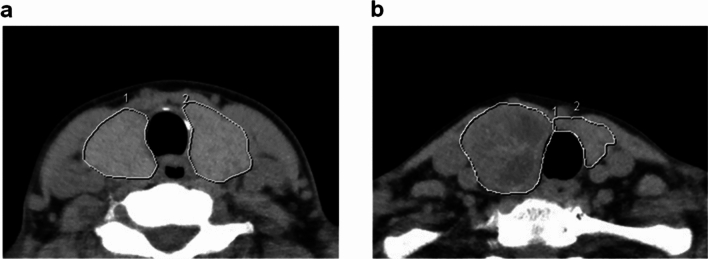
Regions of interest superimposed on the thyroid gland on the computed tomography images.The sum of the thyroid volume obtained from each slice was denoted as the total thyroid volume a Graves’ disease, b Plummer disease
